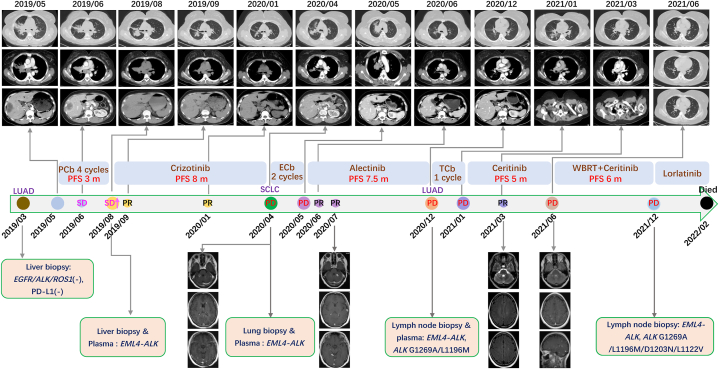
Course of disease with driver mutations, treatment history, and computed tomography images.LUAD, lung adenocarcinoma; SCLC, small cell lung cancer; PCb, pemetrexed and carboplatin; ECb, etoposide and carboplatin; TCb, paclitaxel and carboplatin; SD, stable disease; PR, partial response; PD, progressive disease; PFS, progression-free survival; WBRT, whole brain radiation therapy.
